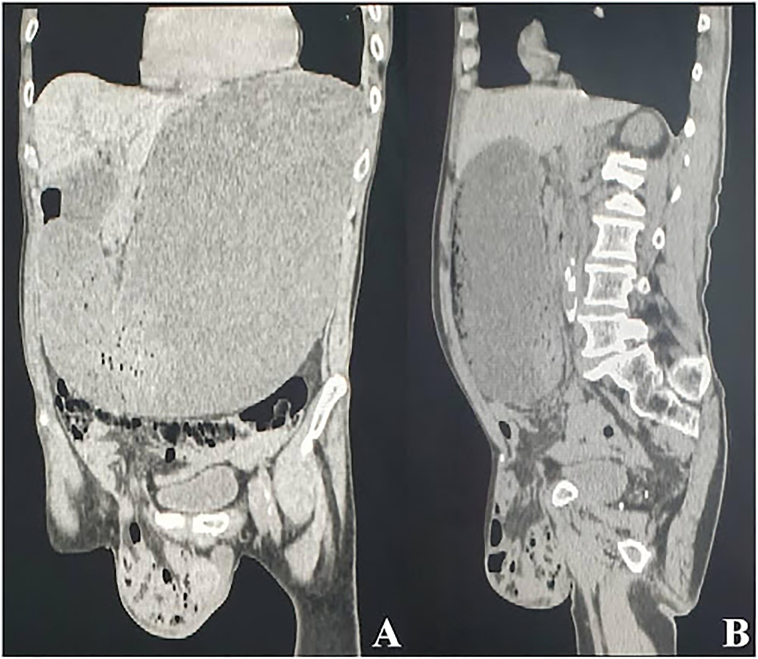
A,B. Preoperative abdominal computed tomography scan revealing massive gastroduodenal dilatation and small bowel loops in the right inguinal hernia sac. Fig. 3 A coronal view, Fig. 3 B sagittal view.
